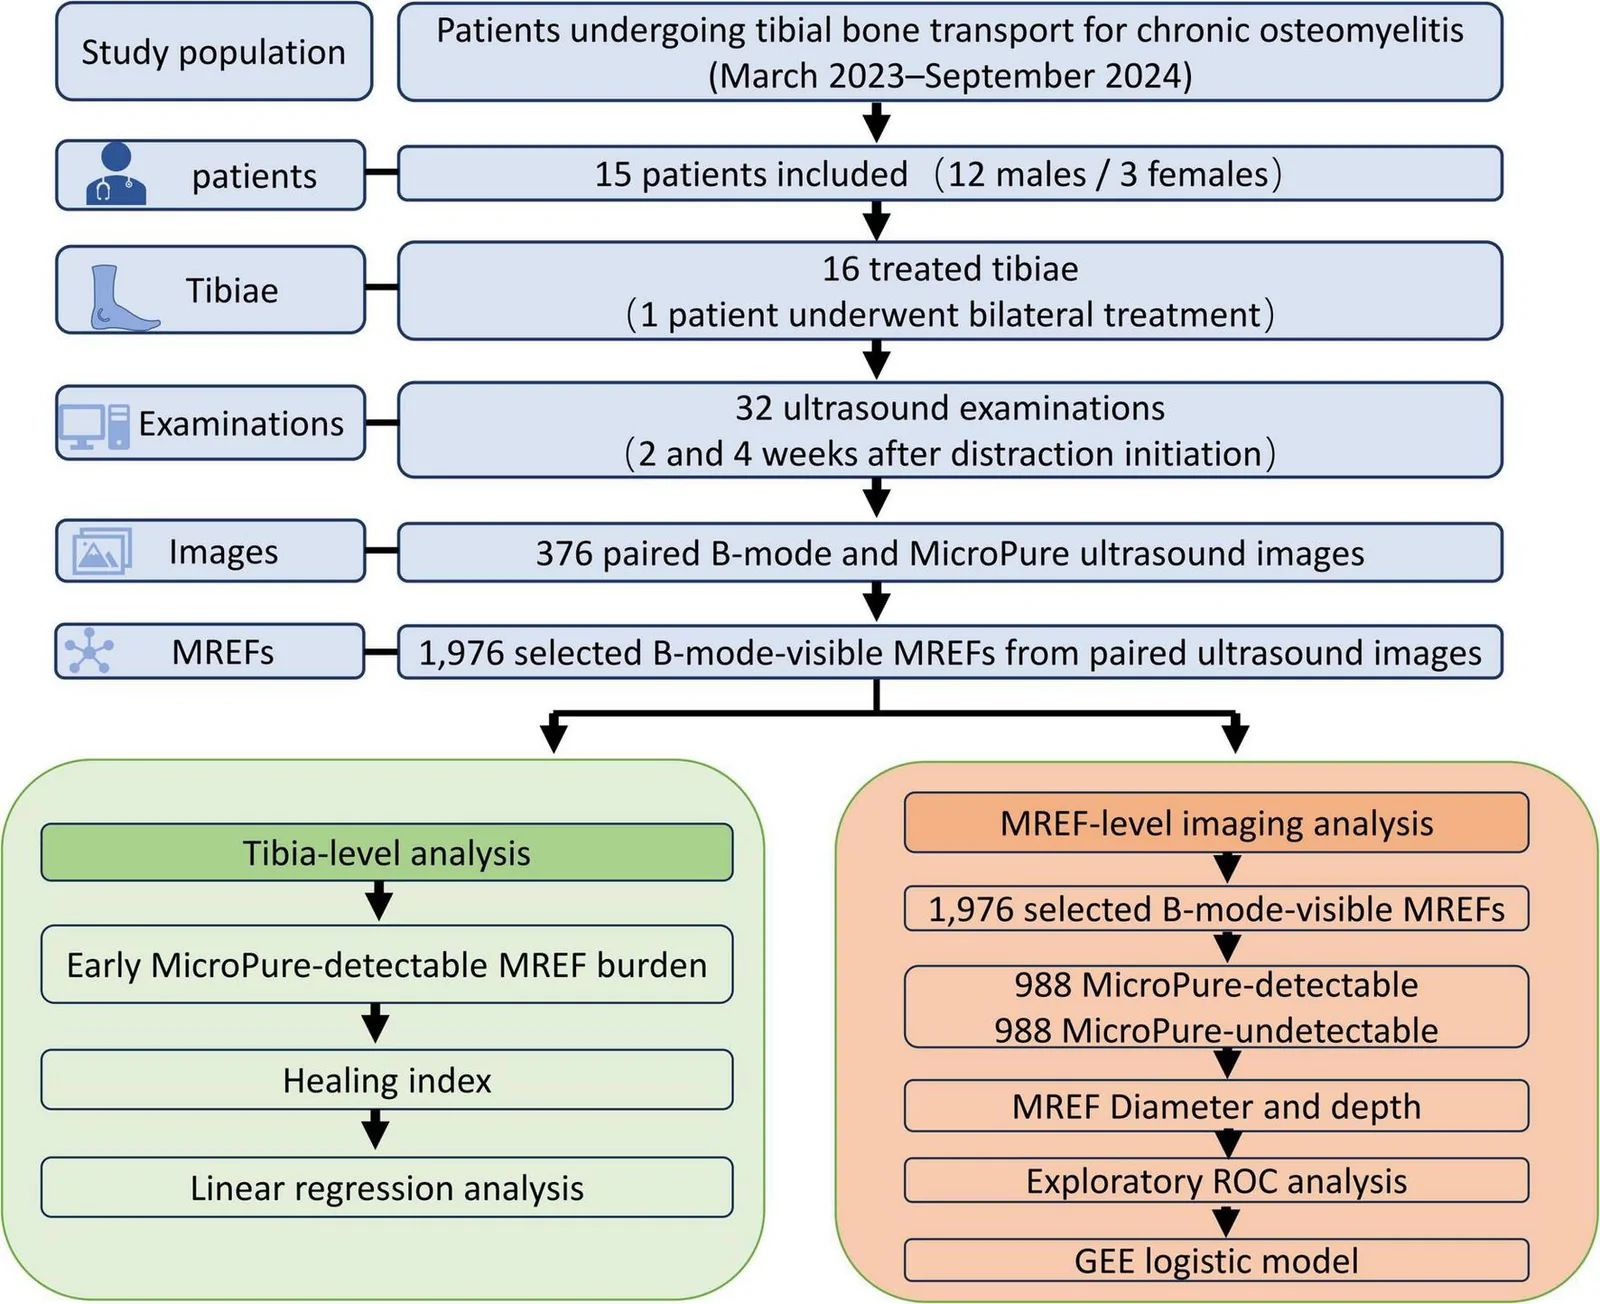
Study design and analytical workflow. Fifteen patients undergoing tibial bone transport for chronic osteomyelitis between March 2023 and September 2024 were included in this retrospective study. A total of 16 tibiae and 32 ultrasound examinations performed at 2 and 4 weeks after distraction were analyzed. B-mode ultrasound and MicroPure imaging were used to evaluate regenerate bone. Two levels of analysis were conducted. Tibia-level analysis assessed the association between early MicroPure-detectable MREF counts and healing index. Foci-level analysis included 1,976 MREFs (988 MicroPure-detectable and 988 MicroPure-undetectable MREFs) and evaluated the associations between MREF diameter, depth, and MicroPure detectability.
